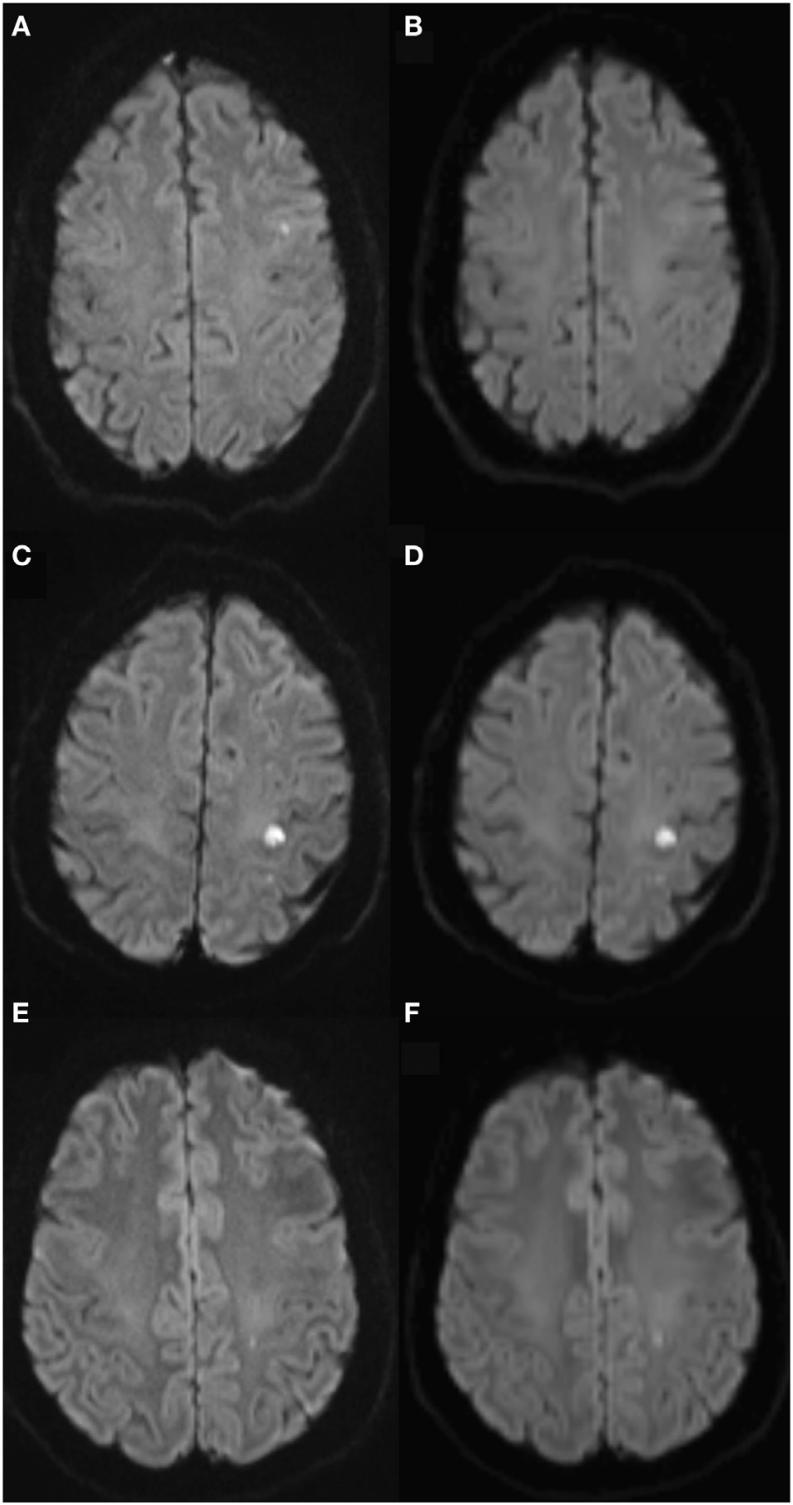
Qualitative lesion brightness on 3- and 20-gradient diffusion imaging. Diffusion-weighted imaging (DWI) lesion shows on 3-gradient DWI (3DWI) (A), 20-gradient DWI (B) shows no certain lesion, but blur. Panels (C,D) equal lesion brightness on 3DWI (C) and 20DWI (D). Panels (E,F) lesion brightest on 20DWI (F).
